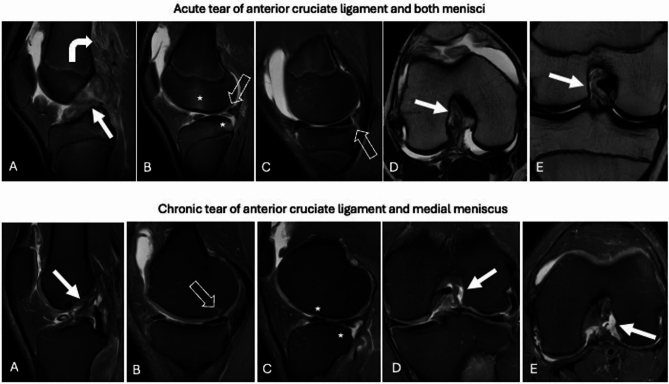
Acute tear of anterior cruciate ligament and both menisci. Knee MRI performed 2 days after trauma shows edematous and thickened appearance of the anterior cruciate ligament with focal irregularity of the bundles (white arrows) on sagittal fat-suppressed proton density weighted (A), axial (D) and coronal (E) T2-weighted images. Indirect signs of acute tear are peri-capsular edema (A; curved arrow) and lateral femoro-tibial bone edema (B; asterisks). Also note the radial tear of the lateral meniscus (B; void arrow) and meniscocapsular separation (C; void arrow). Chronic tear of anterior cruciate ligament and medial meniscus. Knee MRI performed 5 months after trauma shows proximal avulsion and thinning of the anterior cruciate ligament (white arrows) on sagittal (A), coronal (D), and axial (E) fat-suppressed proton density weighted images, without bone edema (C; asterisks). Also note chondral injury of the medial femoral condyle (B; void arrow) and medial meniscus tear (B)
